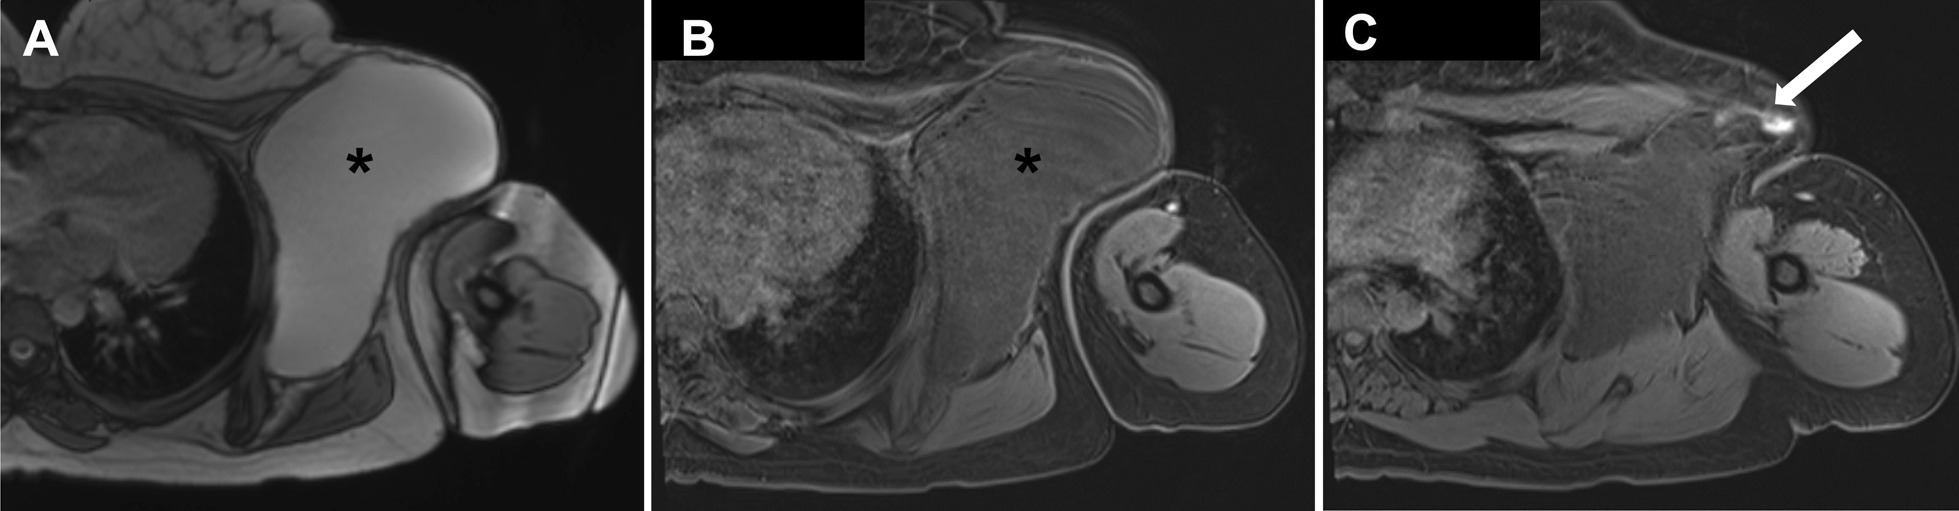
Axial images from MRI lymphangiogram. A Noncontrast steady-state free precession demonstrating large cystic mass centered in the left axilla (*). B Ten-minute post contrast image following subcutaneous injection of contrast into the web space of the hand, demonstrating no enhancement of the mass (*). C Post-contrast image demonstrating enhancement of an axillary lymph node overlying the mass (arrow)
